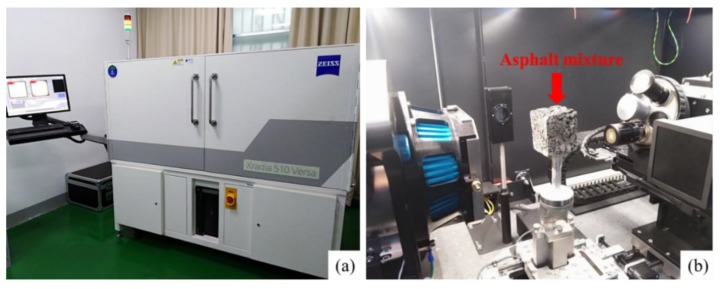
X-ray CT (a) and mixture been scanning (b).
